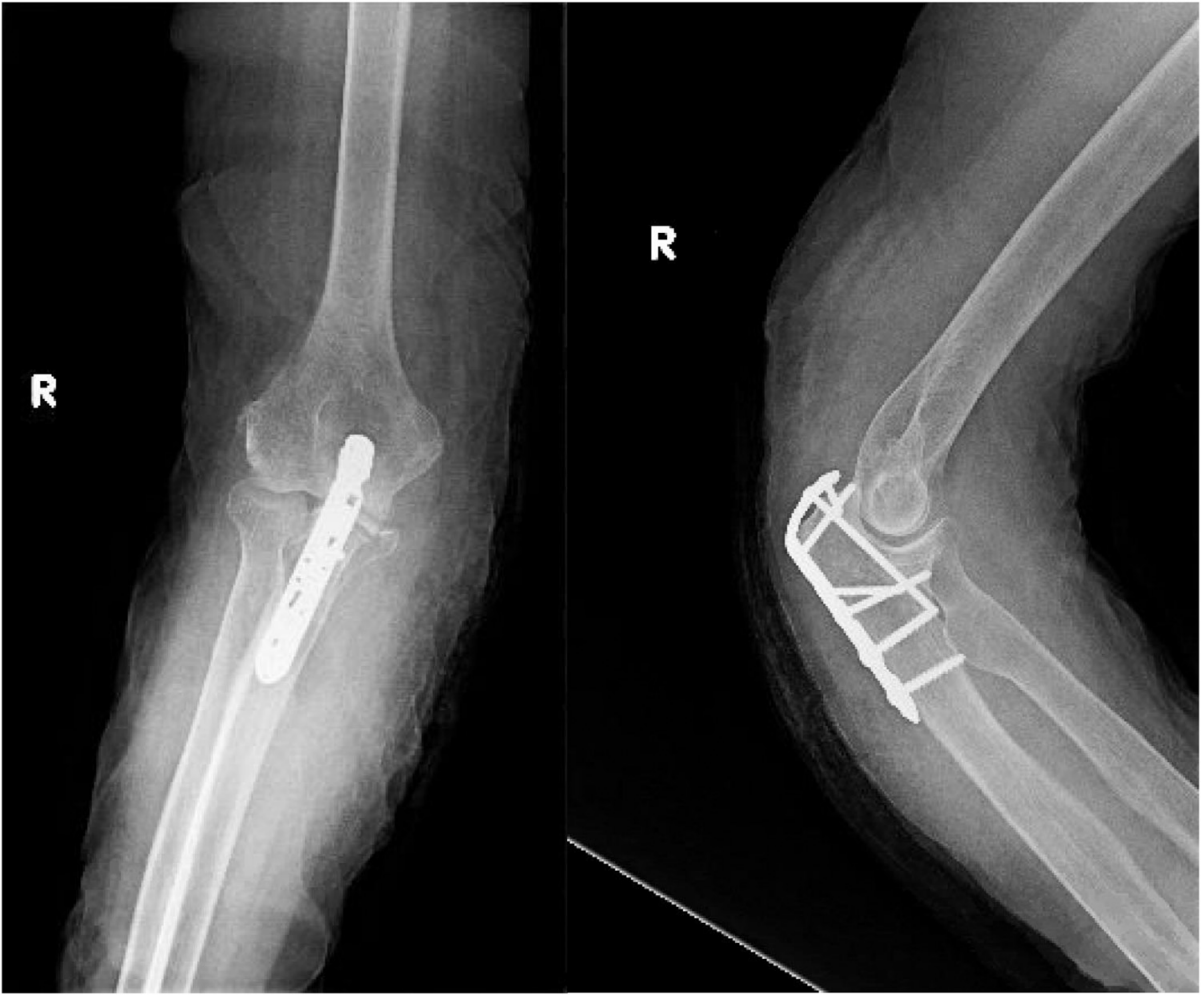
Locked plate fixation for olecranon fracture in a geriatric patient. Postoperative AP and lateral radiographs of an 86-year-old female patient. *AP*, anteroposterior.
